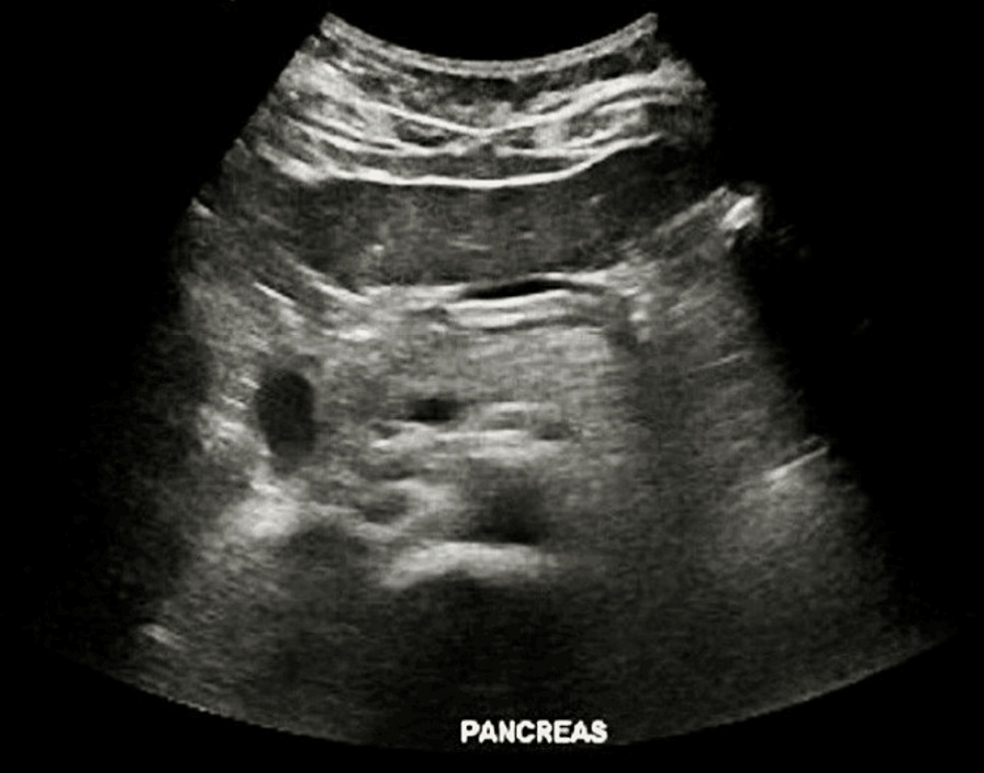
Abdominal ultrasound showing unremarkable gallbladder, no evidence of cholecystitis. Mild diffuse hepatic steatosis
